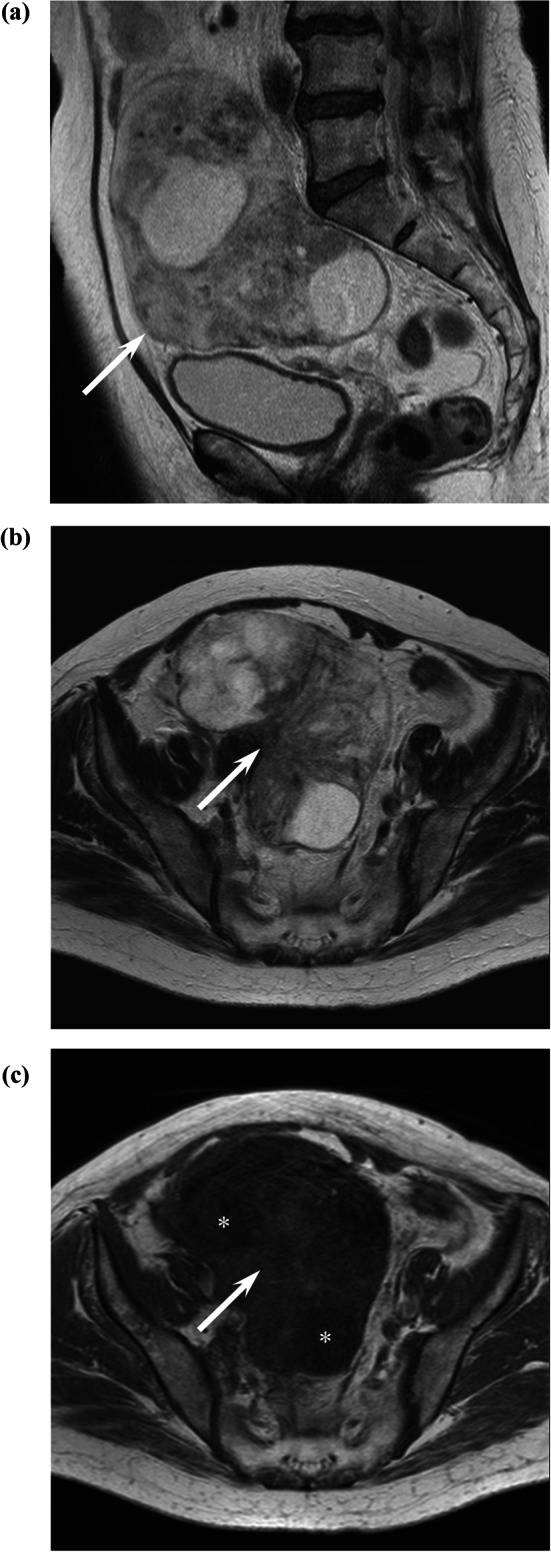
A 65-year-old female with appendiceal mucinous adenocarcinoma. Sagittal (a) and axial (b) T2-weighted images showing a predominantly solid mass (arrow) with cystic components. Axial T1-weighted image (c) showing a solid mass (arrow) with cystic components. The cystic component exhibits low- to iso-signal intensity relative to muscle (asterisk)
